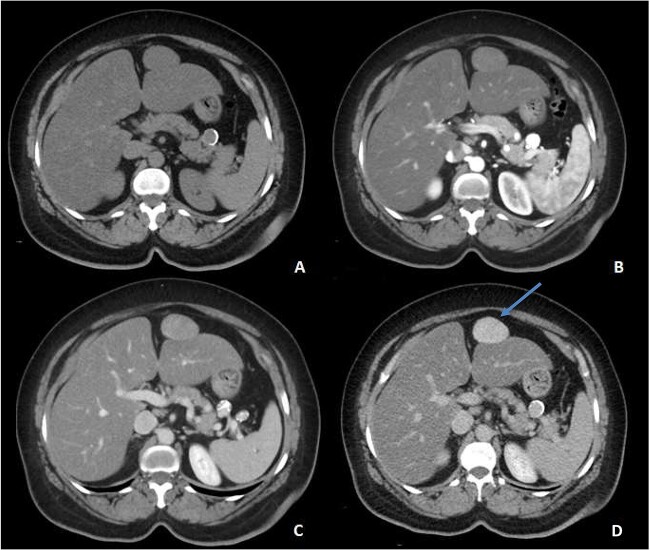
Pre-contrast (A), Arterial (B), Porto-venous (C) and Delayed (D) images of contrast enhanced CT demonstrating a well-defined, homogeneous, progressively enhancing mass anterior to the left hepatic lobe, with homogeneous delayed images (blue arrow). Incidental hepatic steatosis and a small splenic artery aneurysm are present.
